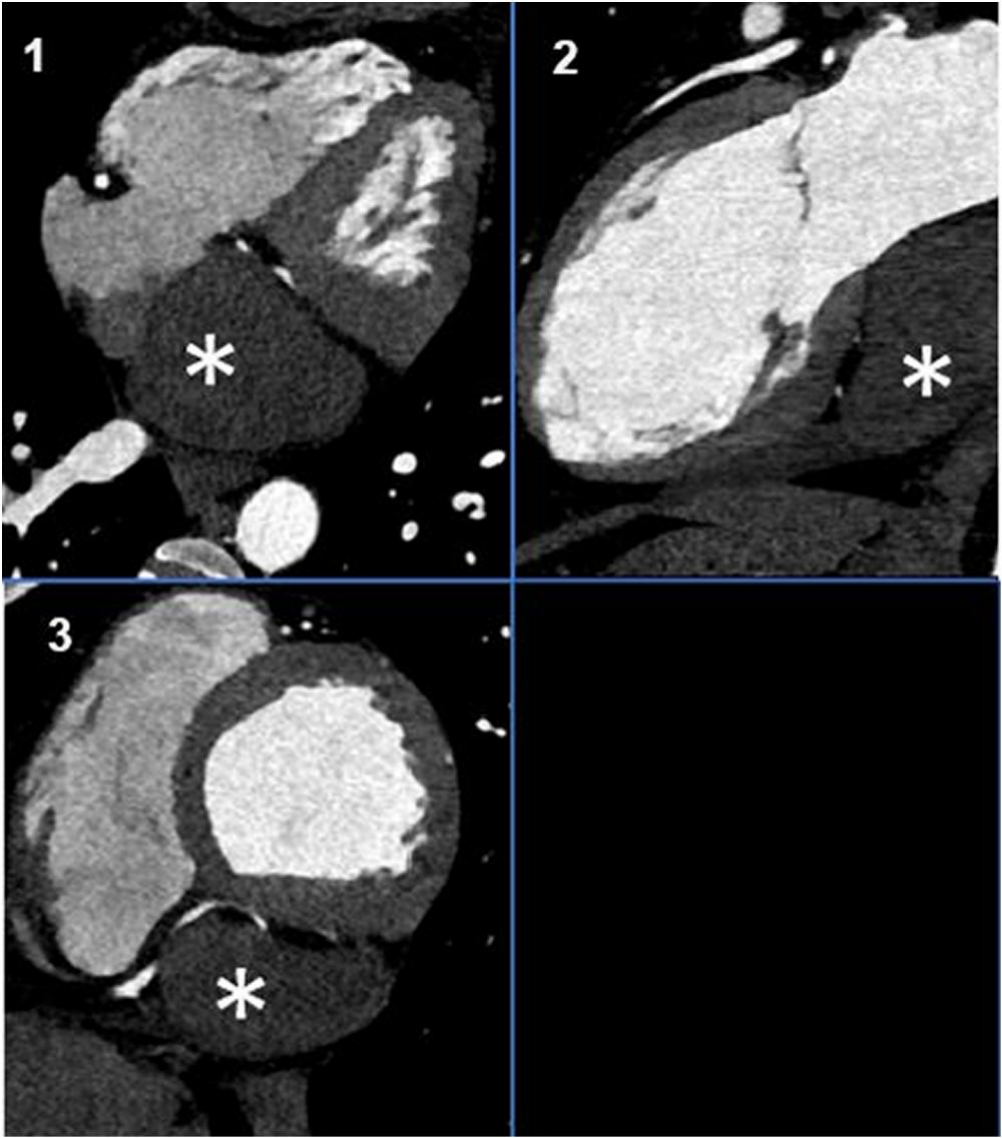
Contrast-enhanced computed tomography angiography of a cardiac cavernous haemangioma measuring 7.5 × 6 × 5 cm (*): The cavernous haemangioma is non-enhancing and appears as a hypodense heterogenous mass presented in different planes. (1) Four-chamber view. (2) Long-axis two-chamber view. (3) Short-axis view.
